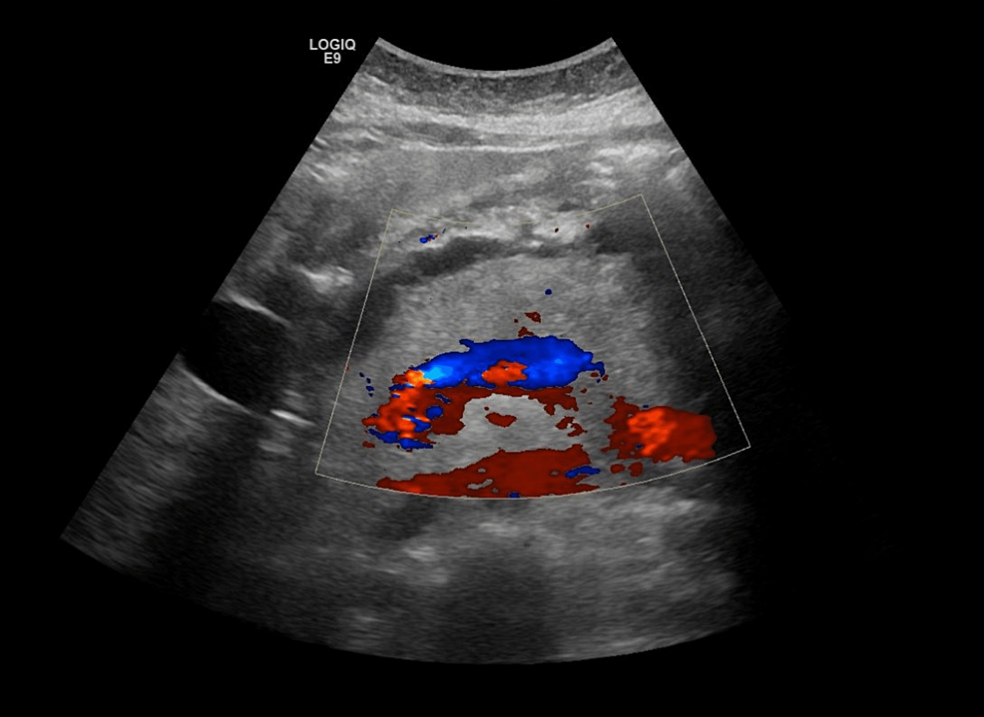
Doppler ultrasound abdomen showed diffusely enlarged pancreas more at the tail with an irregular surface and surrounding peri-pancreatic free fluid suggestive of acute pancreatitis
